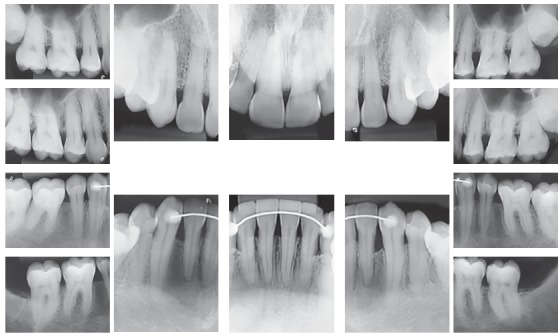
Follow-up periapical radiographs nine years and three months after treatment completion.
